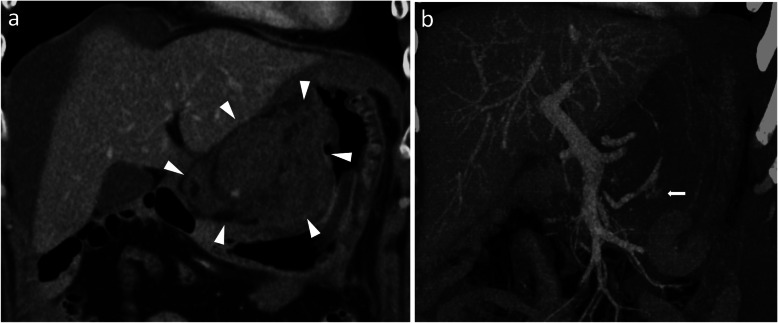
a: Abdominal computed tomography showed a mass on the lesser curvature (white triangles) that showed enhancement in the early phase. b: There was extravasation of the contrast media from the right gastric artery in the late phase (white arrow)
